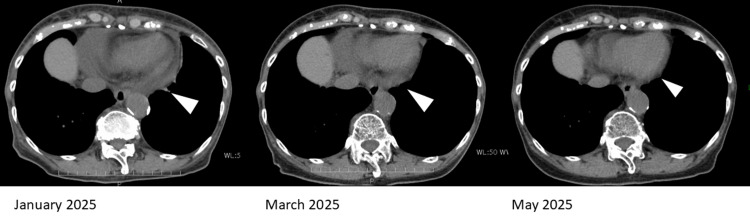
Pericardial effusion on CT (in January 2025: selpercatinib 80 mg/day; March and May 2025: selpercatinib 40 mg/day) Pericardial effusion observed in January 2025 while taking selpercatinib 80 mg/day gradually decreased after the selpercatinib dose was reduced to 40 mg/day (white arrow) CT: computed tomography
